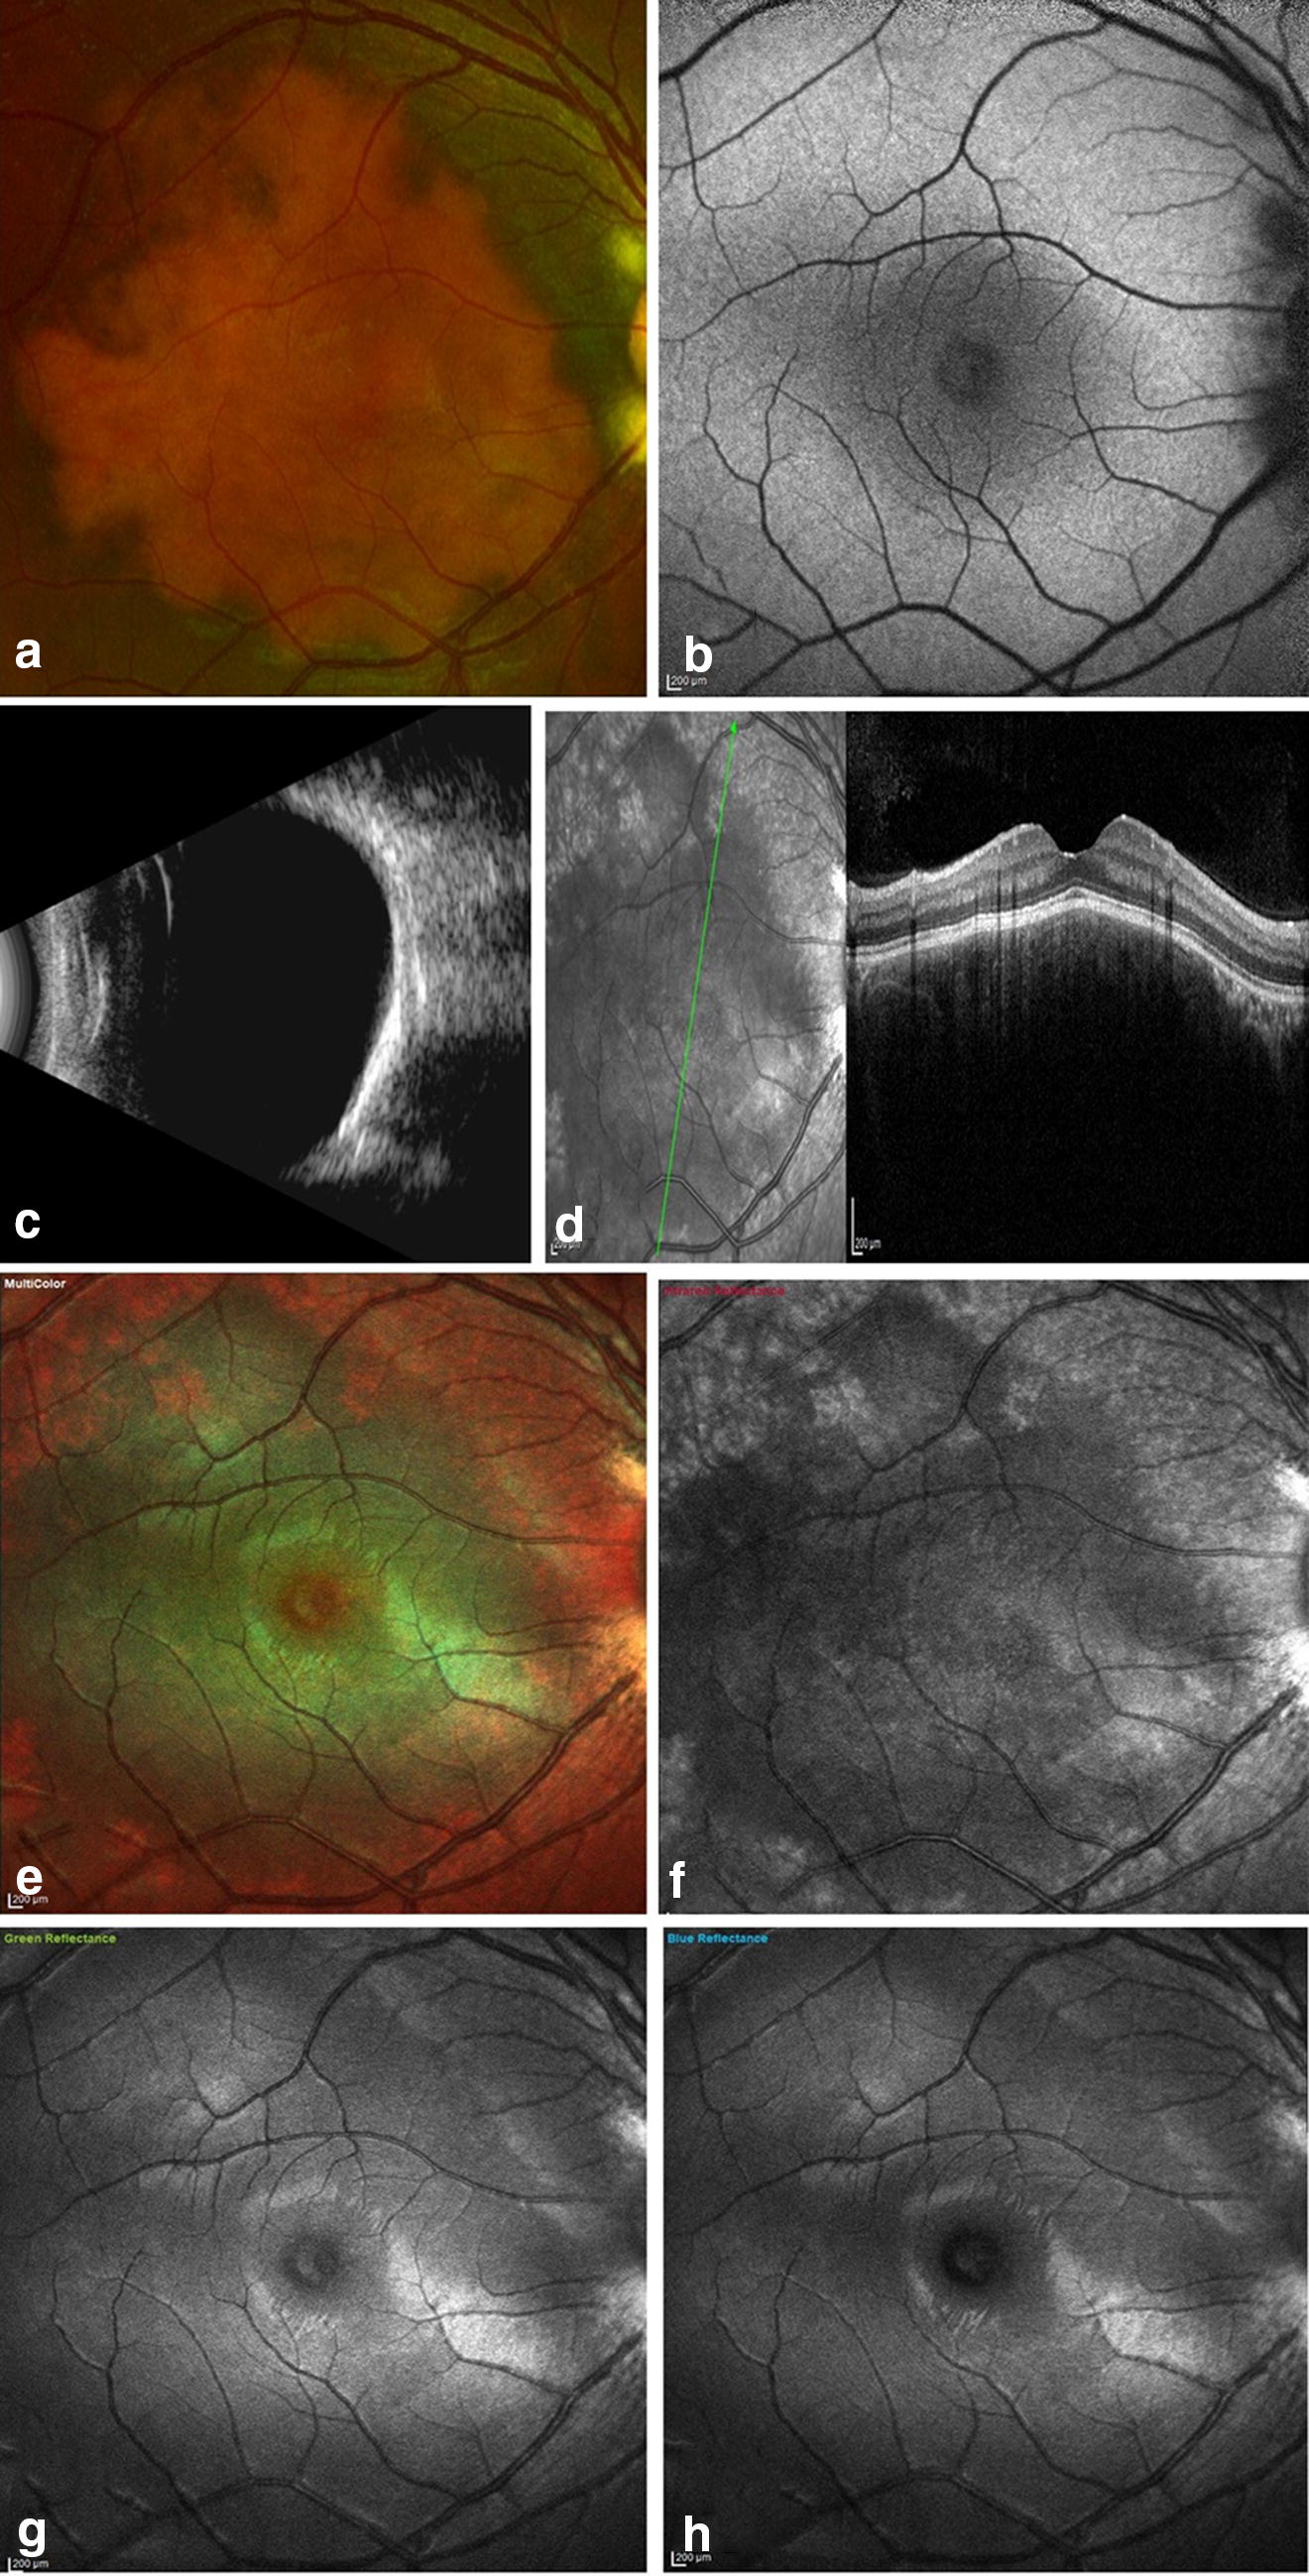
Case 1—Multimodal imaging with color fundus (CF), fundus autofluoroscence (FAF), optical coherence tomography (OCT), ultrasonography (USG) and multicolour (MC) imaging in a patient with RE calcified choroidal osteoma. a CF showing RE orange coloured lesion underneath the retinal pigment epithelium (RPE) at the posterior pole. b FAF showing iso autofluoroscence in the region of interest. c High bone density noted on USG b-scan. d Radial OCT scan passing thru’ the lesion shows retinal elevation with overlying normal retinal contour. e MC image showing the green colouration at the posterior pole. f Infrared reflectance showing dark areas corresponding to the tumour lesion. g, h Green and blue reflectance images do not show any changes due to CO at the posterior pole
